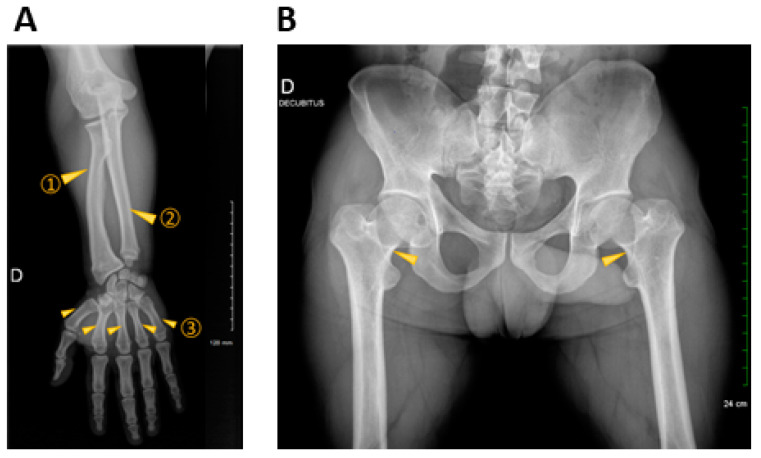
X-ray findings in proband. (A) A standard radiograph of right forearm and hand with shortening of the radius ①, the ulna ②, and the metacarpal bones ③. (B) Standard radiograph of pelvis showing a major shortening of the femoral necks (arrowheads). D: right.
